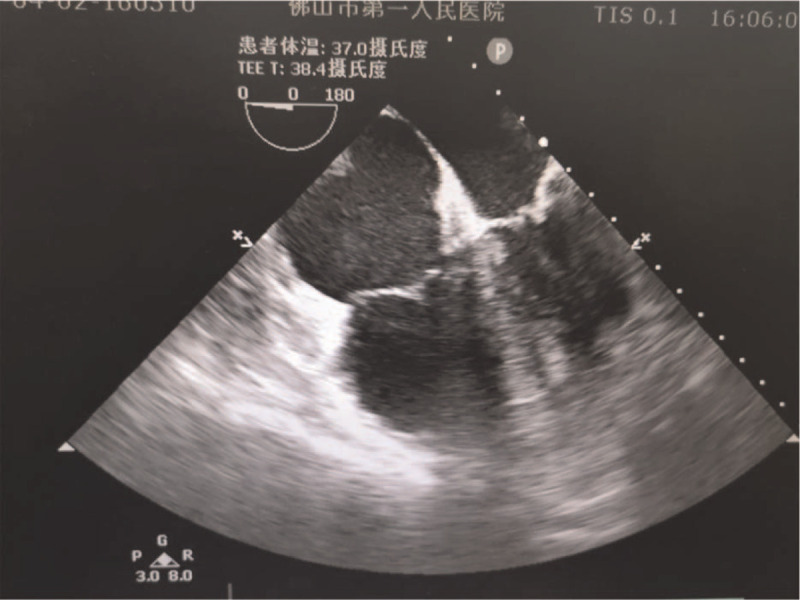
Transesophageal echocardiography showed enlarged right atrium and right ventricle with leftward deviation of the septum and mild-moderate elevation of pulmonary artery pressure, with no significant gas in the cardiac chambers.
